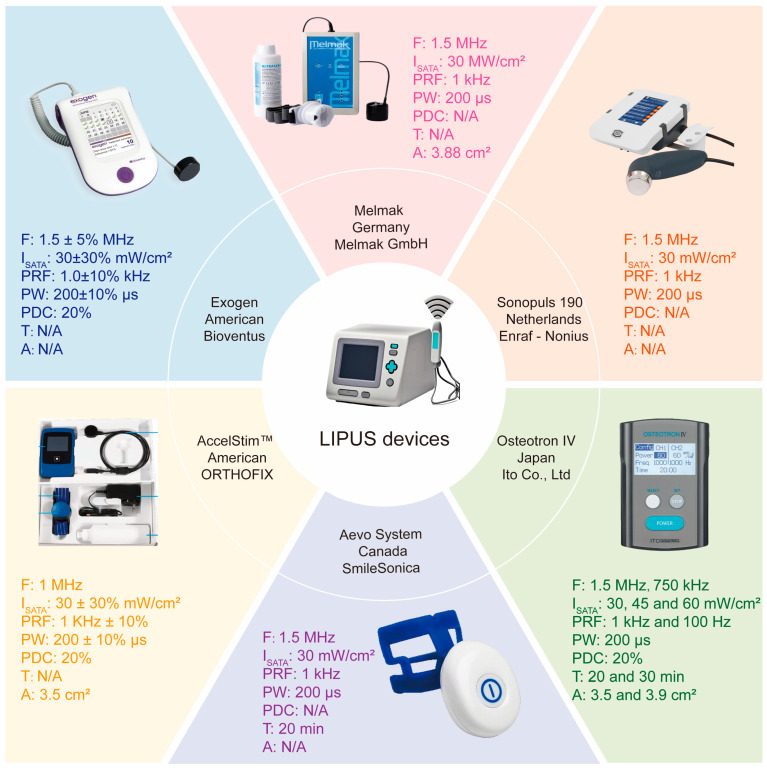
LIPUS devices and their parameters for experimental research and clinical use in different countries. Six LIPUS devices from five countries worldwide are listed, including the device names, the companies they belong to, and their countries of origin. Moreover, the different parameters that each device can modulate are listed in detail. LIPUS, low-intensity pulsed ultrasound; F, frequency; I_SATA_, space-averaged time-averaged intensity; PRF, pulse repetition frequency; PW, pulse width; PDC, pulse duty cycle; T, exposure time; A, area of ultrasonic transducer; N/A, not available or not applicable.
